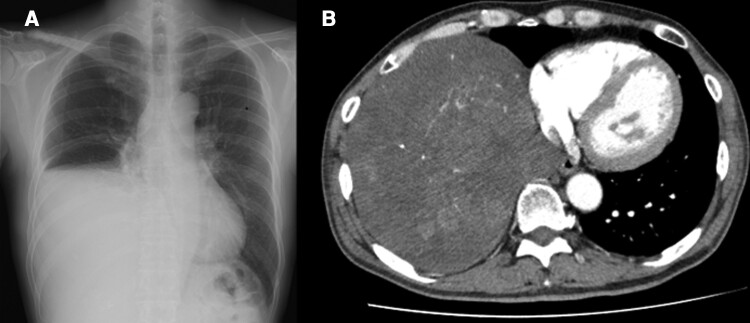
Chest A, x-ray and B, computed tomography (CT) scan. A, Chest radiography displayed a large thoracic tumor measuring 150 × 170 × 120 mm in the right thorax. B, CT scan images showed that tumor was non-homogeneously enhanced by the contrast material.
